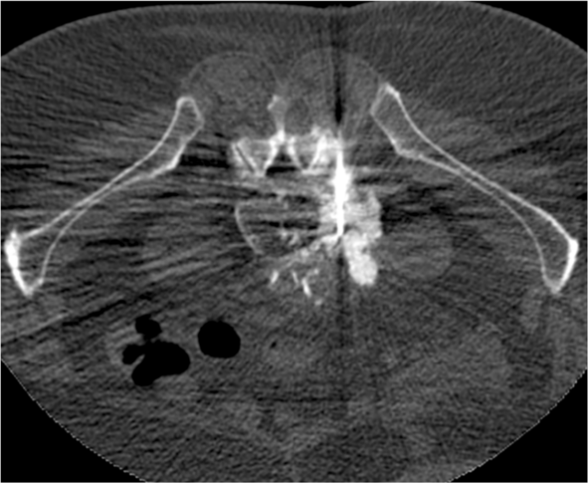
A percutaneous biopsy was performed under CT guidance using a Bonopty 16G biopsy set
